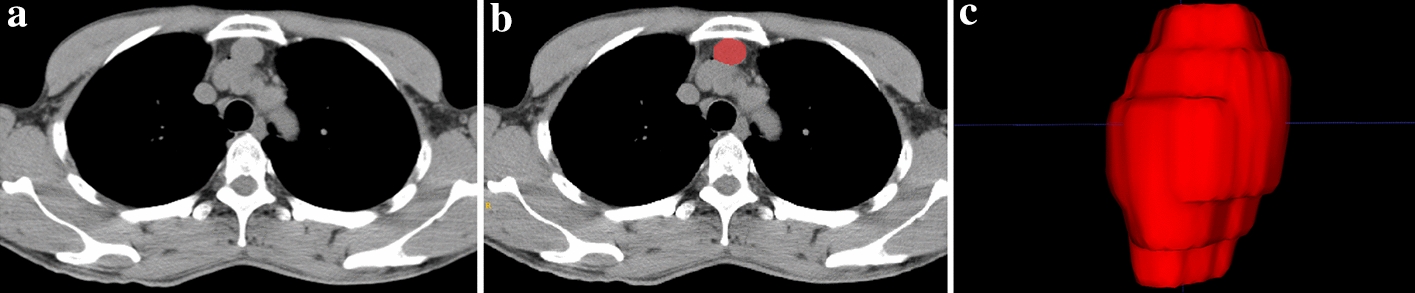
Lesion segmentation. a CT images were acquired first; b radiologists manually draw a region slice-by-slice that encloses the contour of lesion; c lesion segmentation in 3D-VOI
